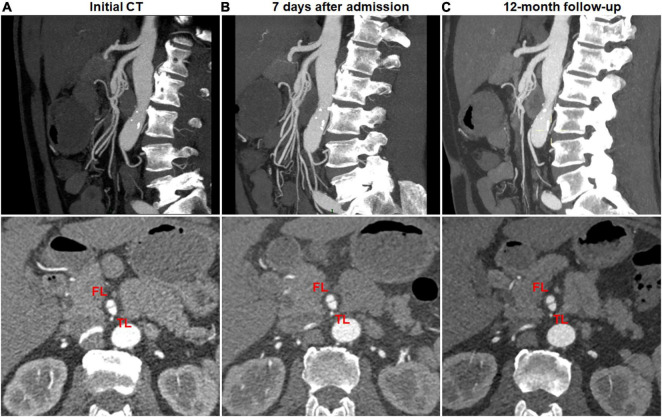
No change of type II spontaneous isolated superior mesenteric artery dissection on follow-up computed tomography (CT) angiography. (A) Initial CT angiography showed the patent “cul-de-sac” shaped false lumen without re-entry in a 66-year-old woman. (B) One week after conservative management, true and false lumens were relatively stable. (C) The true lumen and blind-ending false lumen remained unchanged during the follow-up period of 12 months.
